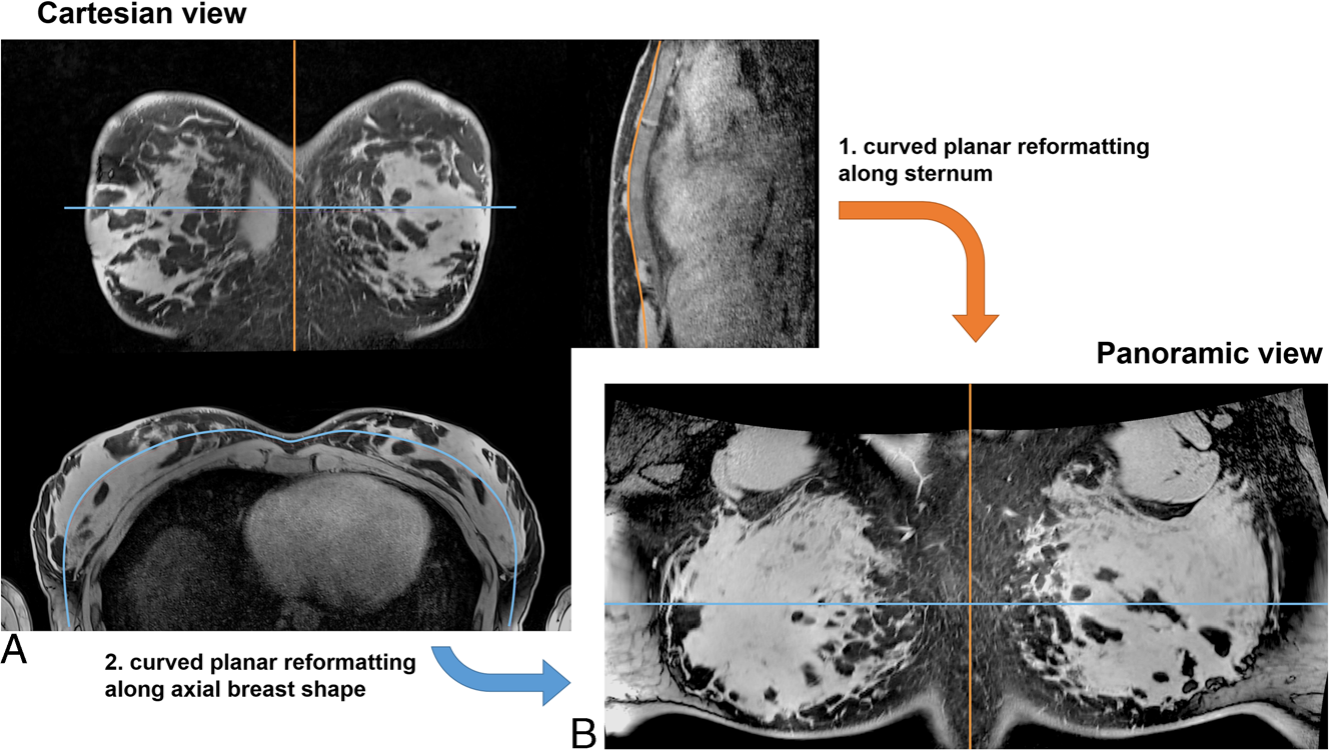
The panoramic breast view. To intuitively visualize both breasts in an efficient manner, a panoramic reconstruction comparable to panoramic dental x-ray was implemented. A, Two lines are manually defined in the Cartesian view: (1) along the sternum (orange) in the sagittal Cartesian view and (2) following the shape of the breast in the axial Cartesian view. The sagittal slice is positioned through the center of the sternum; the axial slice is chosen at the position of the fullest part of the breast. B, The panoramic view is created by consecutive curved planar reformatting along the 2 defined lines.
